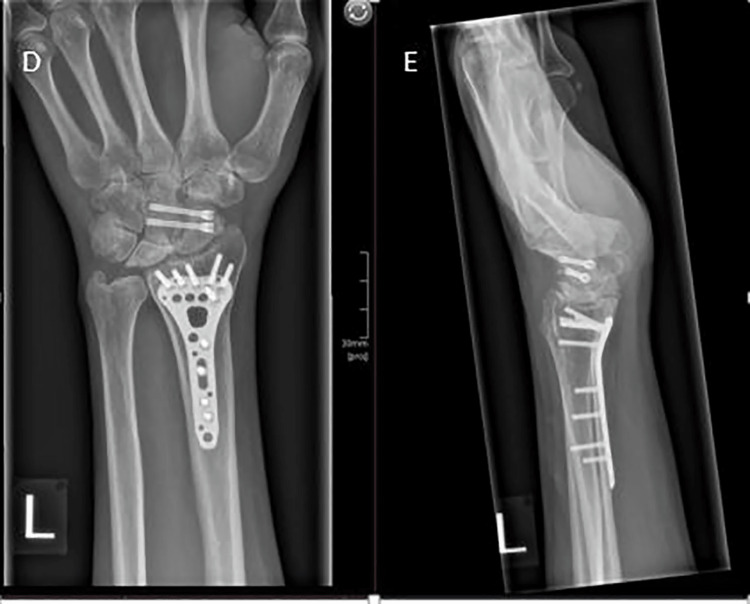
Post-operative anteroposterior (D) and lateral (E) radiographs showing the placement of the SCF screws, fusion of the scaphoid and capitate, and a healed distal radius fracture. SCF, scaphocapitate fusion
